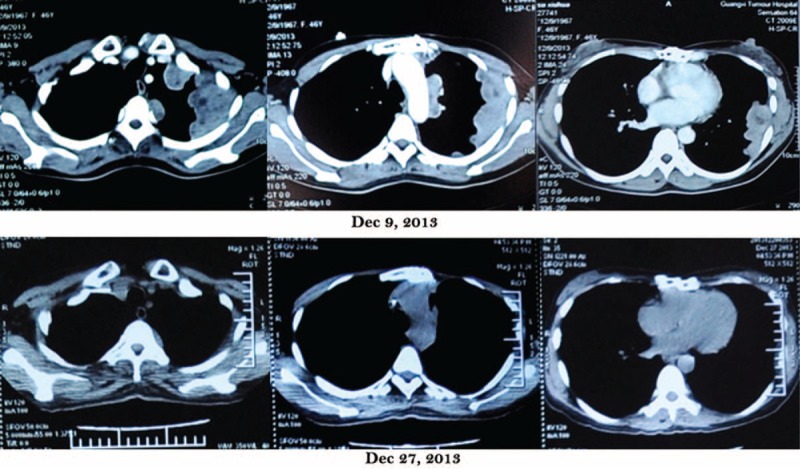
Comparison of CT scan between December 9, 2013, and December 27, 2013. A partial response of tumor following 3 sessions of plasmapheresis was indicated from CT scan. CT = computed tomography.
